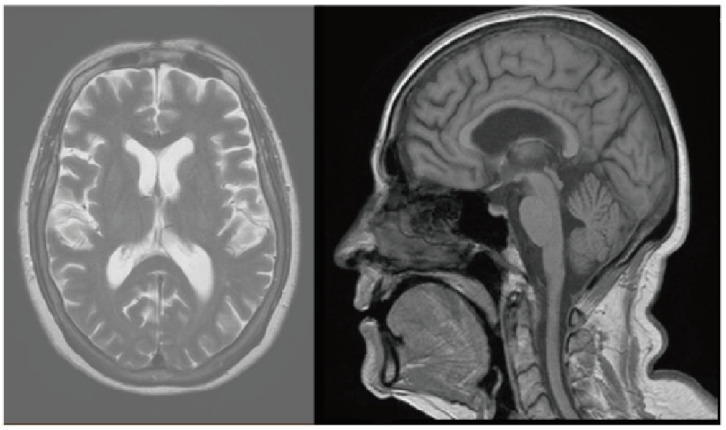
MRI of the head revealed no pathologic findings or evidence of metastasis.
